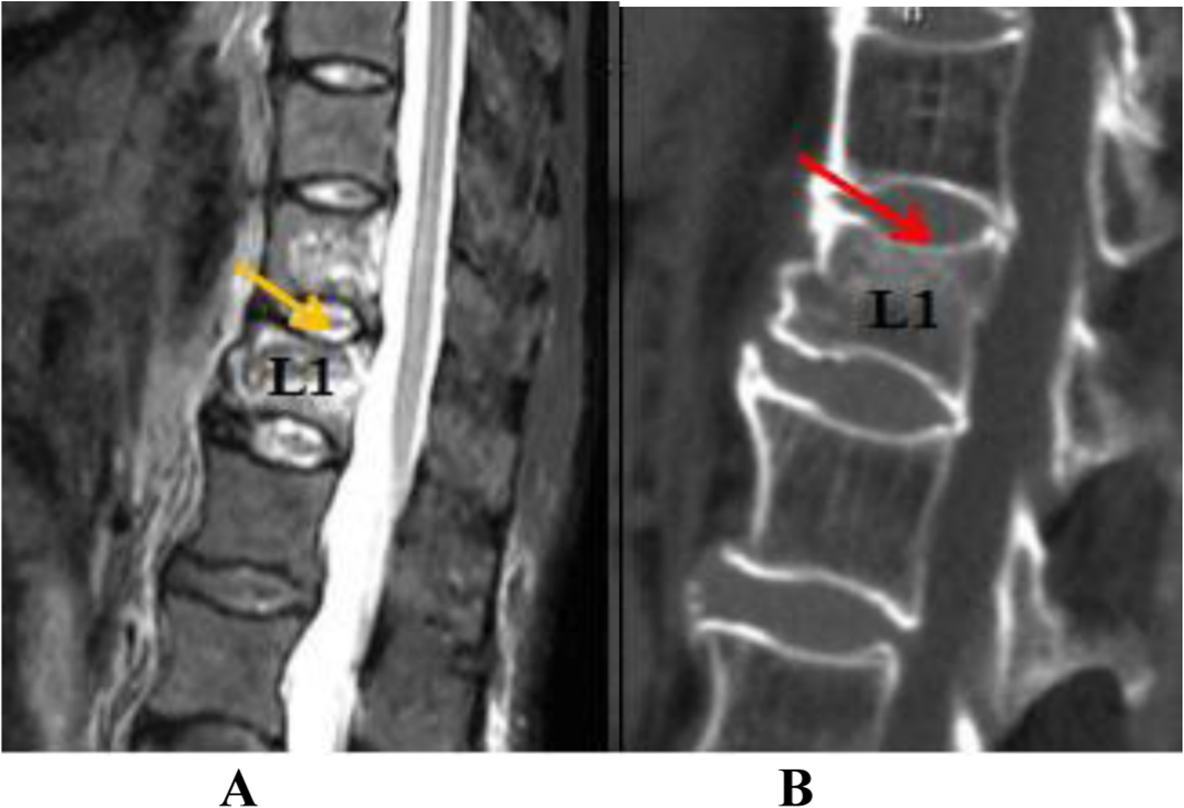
A 73-year-old woman with OVF at level L1. Panel a is an MRI STIR image. The yellow arrow indicates a diffuse high signal in the IVD on the cranial side of the fractured vertebral body. Panel b is a CT image of the fractured vertebral body. The red arrow indicates a linear fracture of the upper endplate of the L1 vertebral body, but there is no sign of displacement or collapse of the endplate. This is a grade 2 EDC injury as delineated in this study
